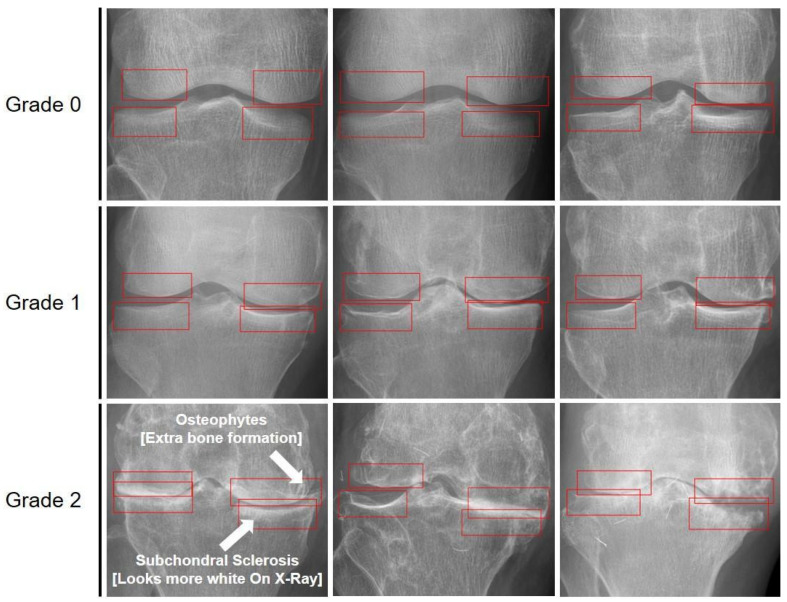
Subchondral labeling and images for each sclerosis grade. The red squares in the image highlight the ROI where subchondral sclerosis is most prominent. Grade 0: no abnormalities in the subchondral bone. Grade 1: mild sclerosis or partial destruction may be observed on the surface of the subchondral bone. Grade 2: significant sclerosis is present, with substantial destruction in multiple areas, potentially impacting joint function. The arrows in the grade 2 image indicate the areas of subchondral sclerosis and OA. These points represent the key lesions characteristic of grade 2 in this study, typically observed in more advanced stages of OA. Notably, subchondral sclerosis appears as white areas in X-ray images.
